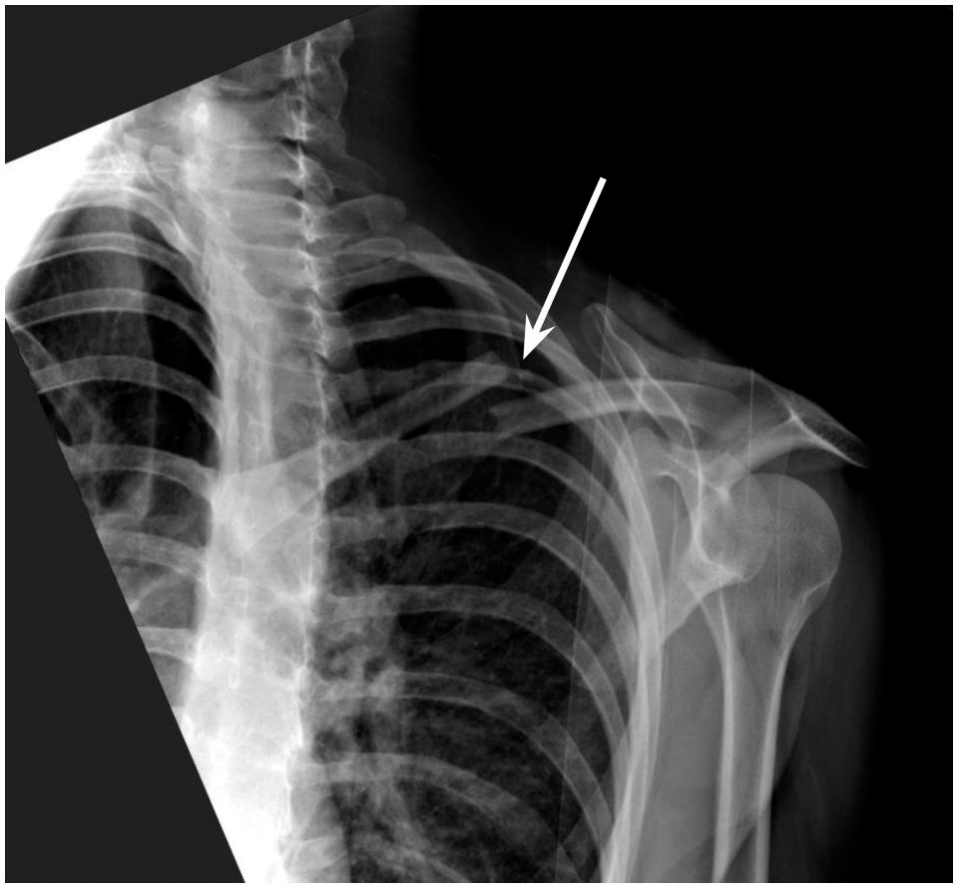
An anterior-posterior radiograph of the left shoulder and clavicle of a 30-year-old woman. A markedly displaced fracture of the clavicle (white arrow) was missed by the AI tool. The fracture was detected by 11 readers in the unaided session and 14 readers in the aided session.
